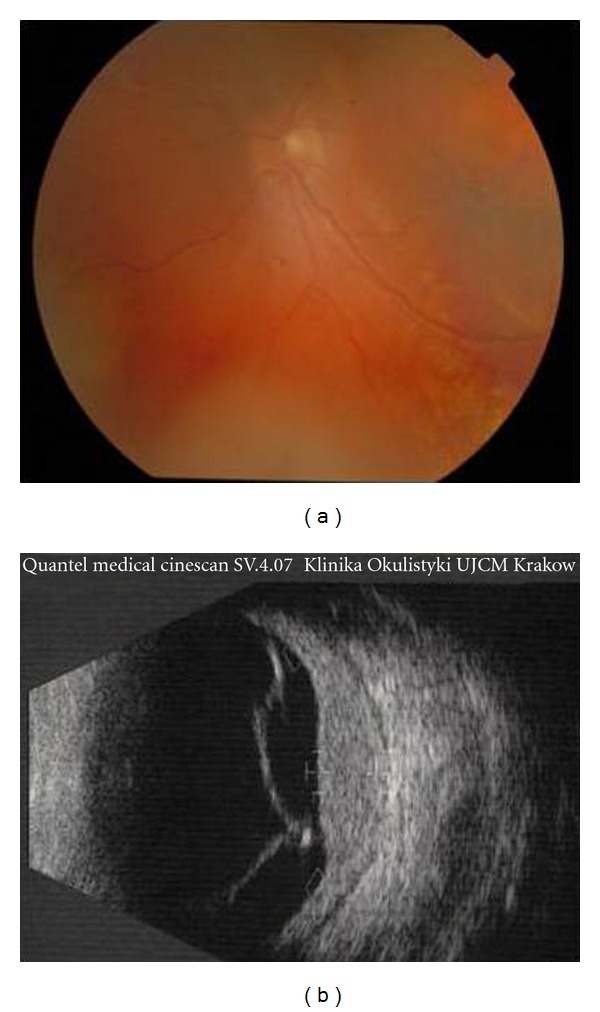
Fundus and B-scan ultrasonography demonstrating diffuse choroidal hemangioma with extensive exudative retinal detachment.
